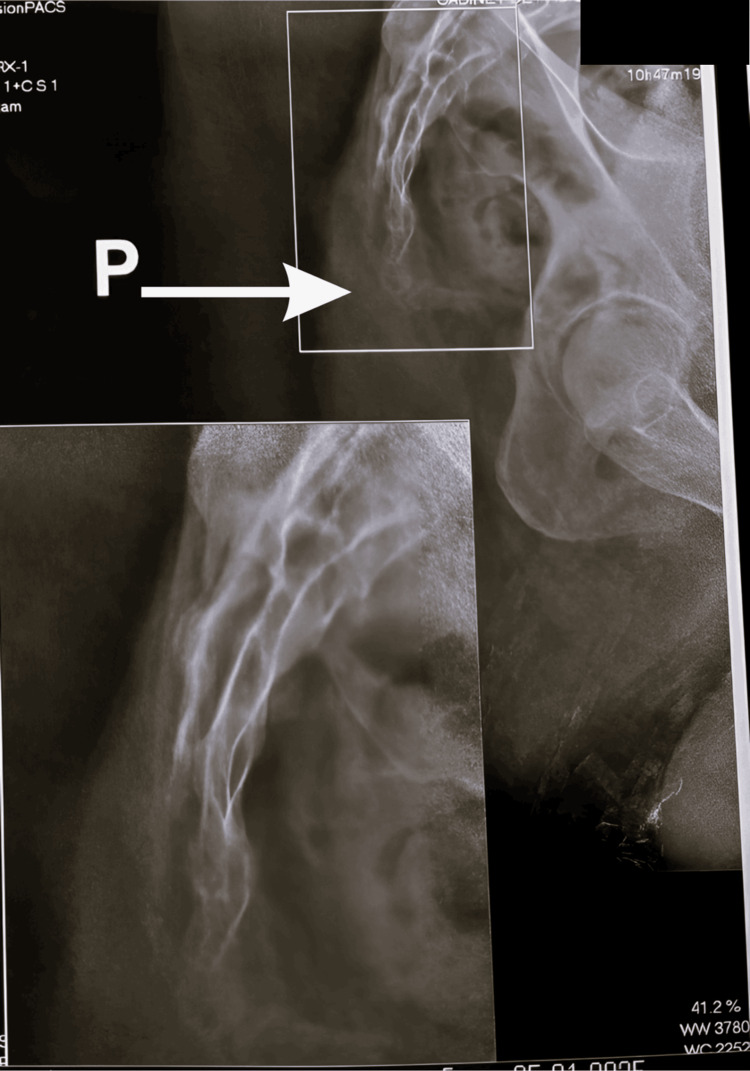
Lateral pelvic radiograph showing anterior angulation of the coccyx The radiograph demonstrates anterior angulation of the coccyx (white arrow), consistent with the patient’s post-traumatic coccydynia.
